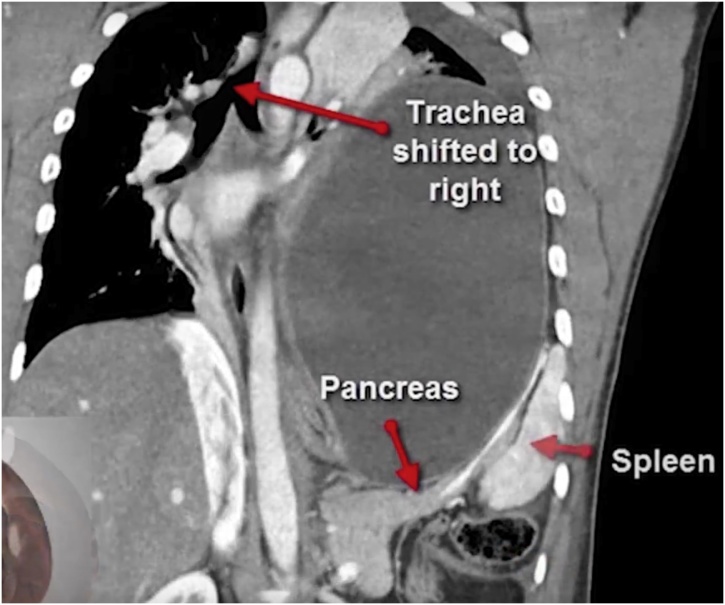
CT showing intra-thoracic transverse colon and pancreas.
